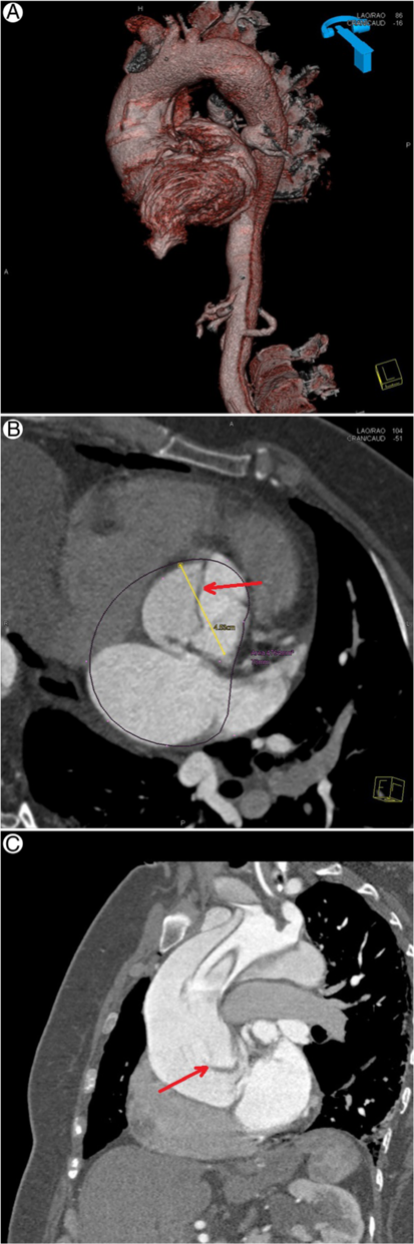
Computed tomography showing dilated ascending aorta with flap of proximal dissection. A. Computed tomography – 3-D reconstruction image: dilated ascending aorta is visible with a flap of proximal dissection along the distance of visualized vessel. B. Computed tomography – transverse plane zoom image: at the level of aortic root a true and false lumina of the vessel are visible, separated by a flap of dissection. C. Computed tomography – tangenital plane: showing ascending aorta and partially false lumen with an intimal flap of proximal aortic dissection.
